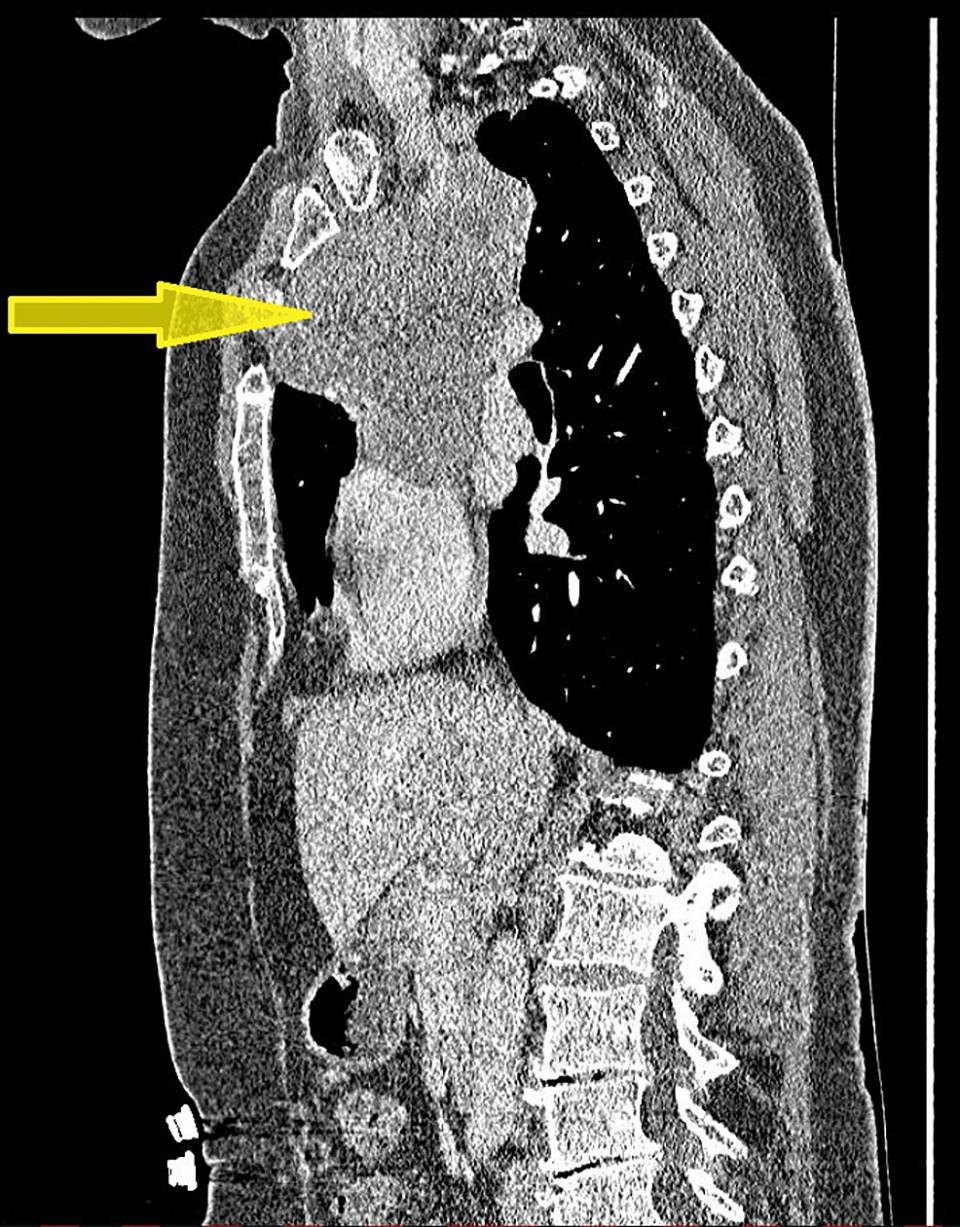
Sagittal-view CT scan showing the anterior mediastinal mass compressing the lower superior vena cava (SVC).
